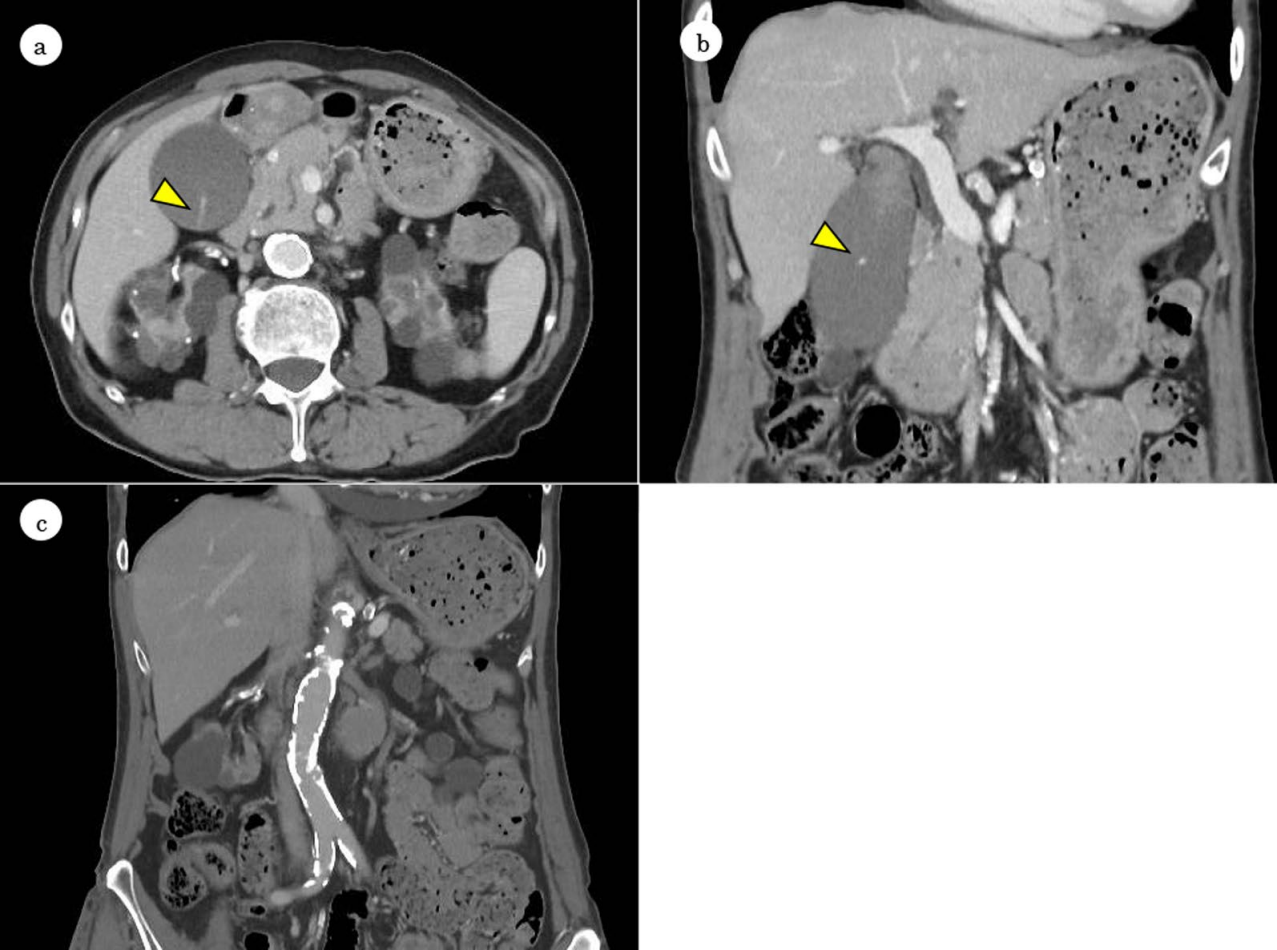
Contrast-enhanced computed tomography scans showing slightly hyperdense material and active extravasation (arrowhead) within a distended gallbladder lumen. There is no evidence of aneurysm of the cystic artery, gallstones, or ascites (a, b). Severe calcification is present in the aorta and the peripheral arteries (c)
